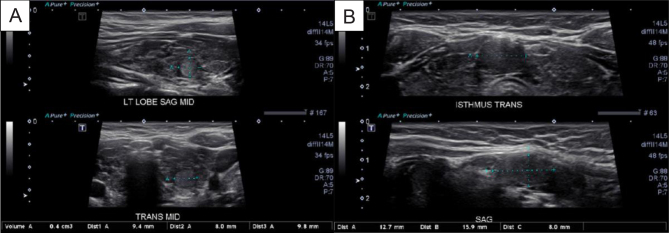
Thyroid ultrasound of two newly present thyroid nodules, a left thyroid nodule (A) and an isthmus nodule (B), that were not present 3 years beforehand.
